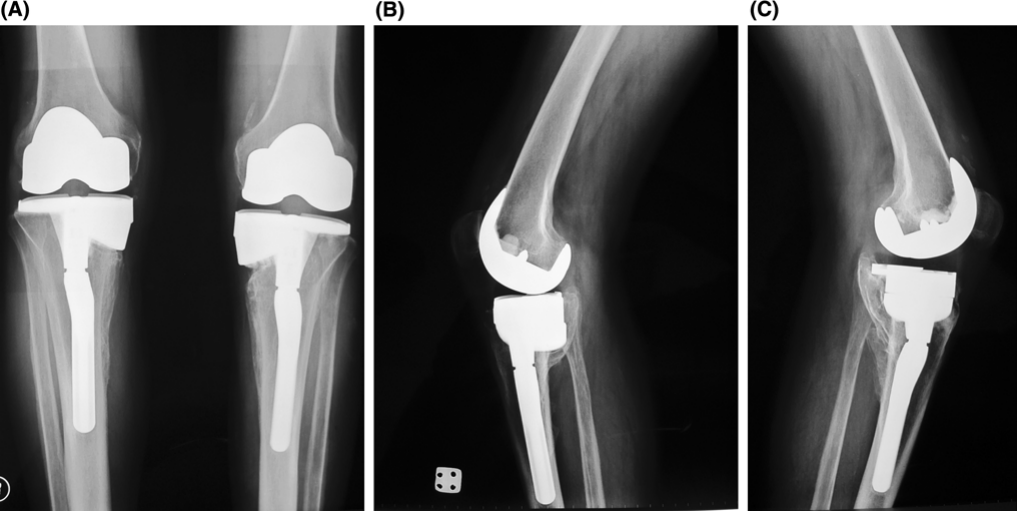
Last control radiographs of both knees. a Anteroposterior view of both knees, b lateral view of right knee, c lateral view of left knee
